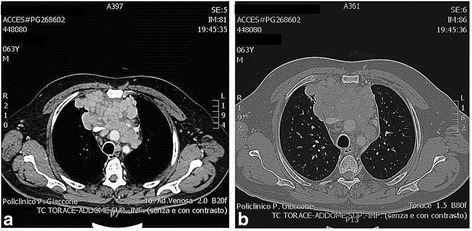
Chest contrast-enhanced computed tomography scan. a Mediastinal window and b lung window: large mediastinal mass (axial: 9.7 × 5.7 cm; longitudinal: 8 cm) formed by multiple confluent lymph nodes with a heterogeneous contrast enhancement that involves the anterior-middle mediastinum. The mass is compressing the jugular vein and the superior cava vein
